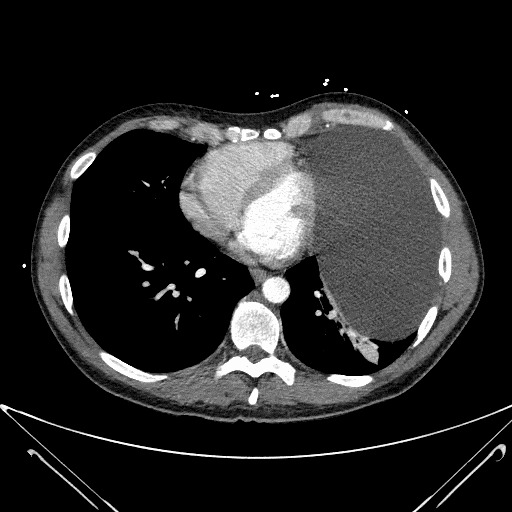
A computed tomography angiogram revealing the large pericardial cyst in an axial cut outlined by the arrows.
